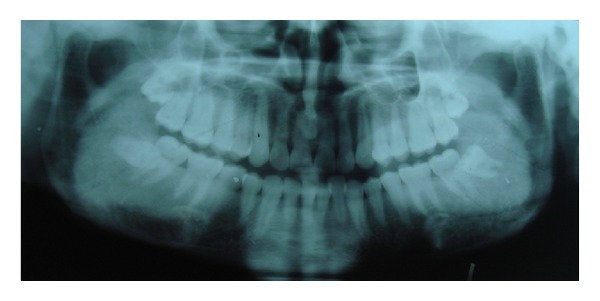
Panoramic radiograph of the patient's brother.
